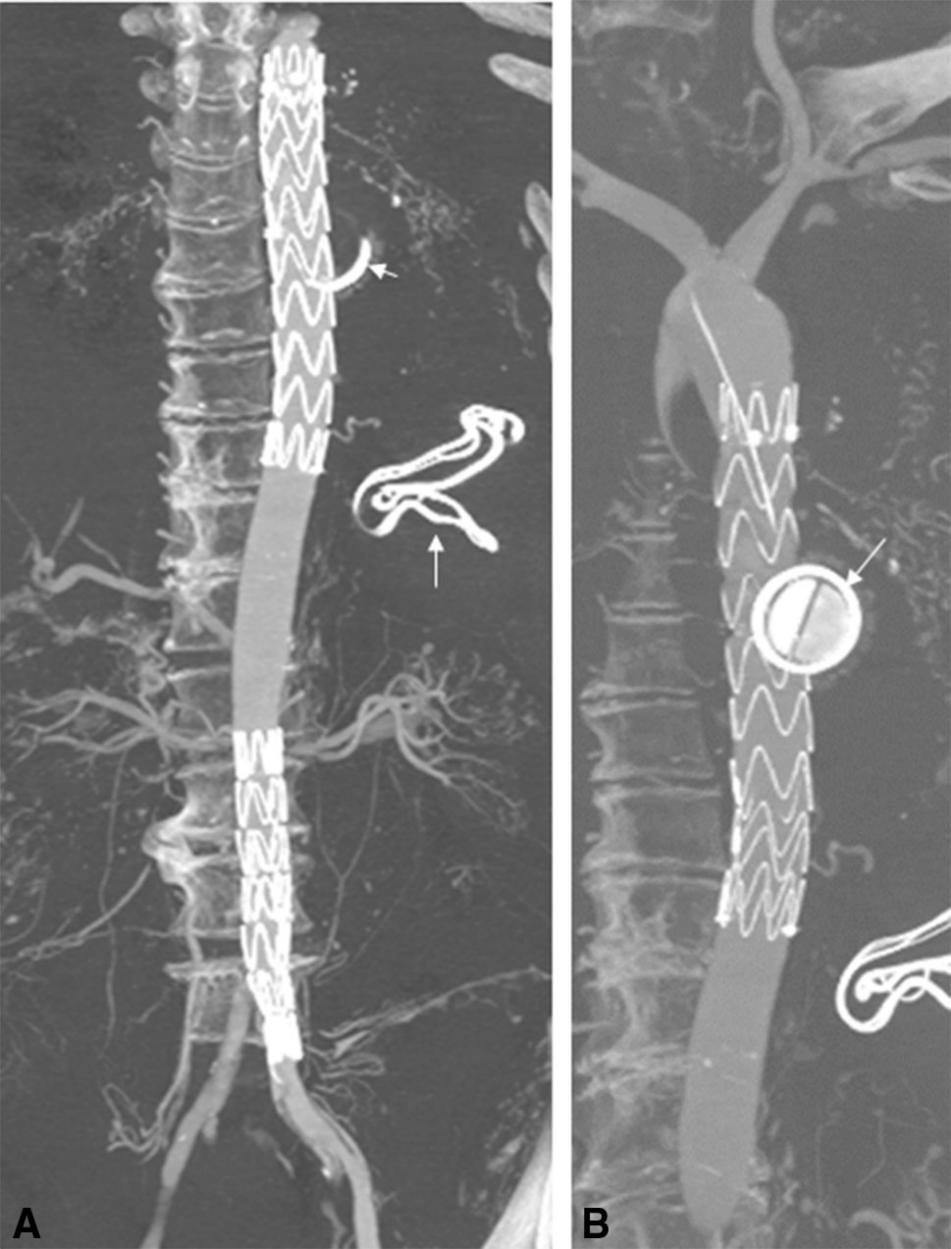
Post-procedure CT scan. CT angiogram (Table 1), MIP 3D reconstruction, allowing vessel and device evaluation (*arrows* point at post-mortem devices unrelated to this work). A Thoracic stent and abdominal stent conducted under fluoroscopic guidance, B thoracic stent in situ
